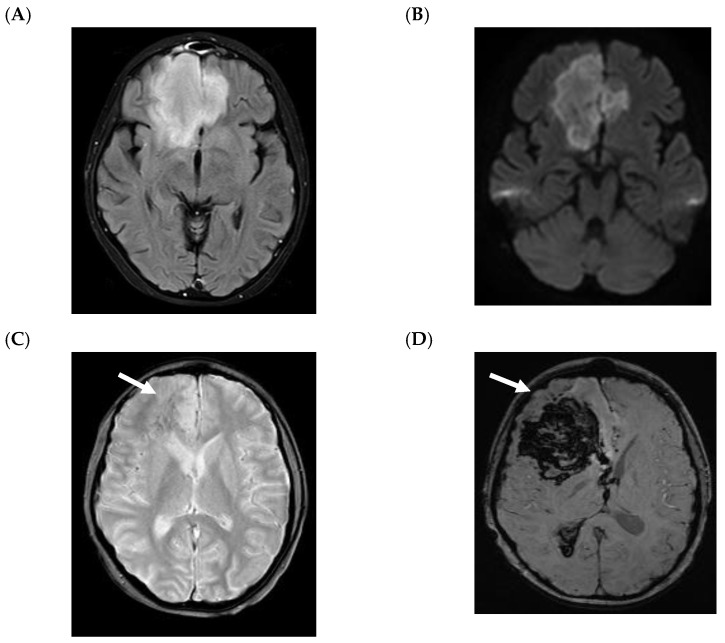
A 12-year-old boy with acute lymphoblastic leukemia with frontal cerebritis in the right and left frontal lobe and probable central nervous system (CNS) invasive mold infection. *Aspergillus fumigatus* and *Rhizopus arrhizus* were isolated outside the CNS. The patient is alive with residual left-sided hemiparesis. A, B and C: Imaging performed during the early stage of frontal cerebritis, mainly on the right side. Fluid-attenuated inversion recovery (FLAIR) image shows hyperintense white matter of frontal lobe (A). Diffusion-weighted image (DWI), b = 1000 s/mm^2^, shows diffusion restriction in the area of frontal cerebritis (B). T2* image (C) shows an ill-defined hypointense area (arrow). No enhancement was seen. After 20 days, magnetic resonance imaging was performed after clinical worsening and suspected vascular involvement (D). Susceptibility-weighted image (SWI; D) shows marked decreased signal intensity and blooming with mass effect. Findings were consistent with major secondary hemorrhage (D, arrow) and intraventricular bleeding.
